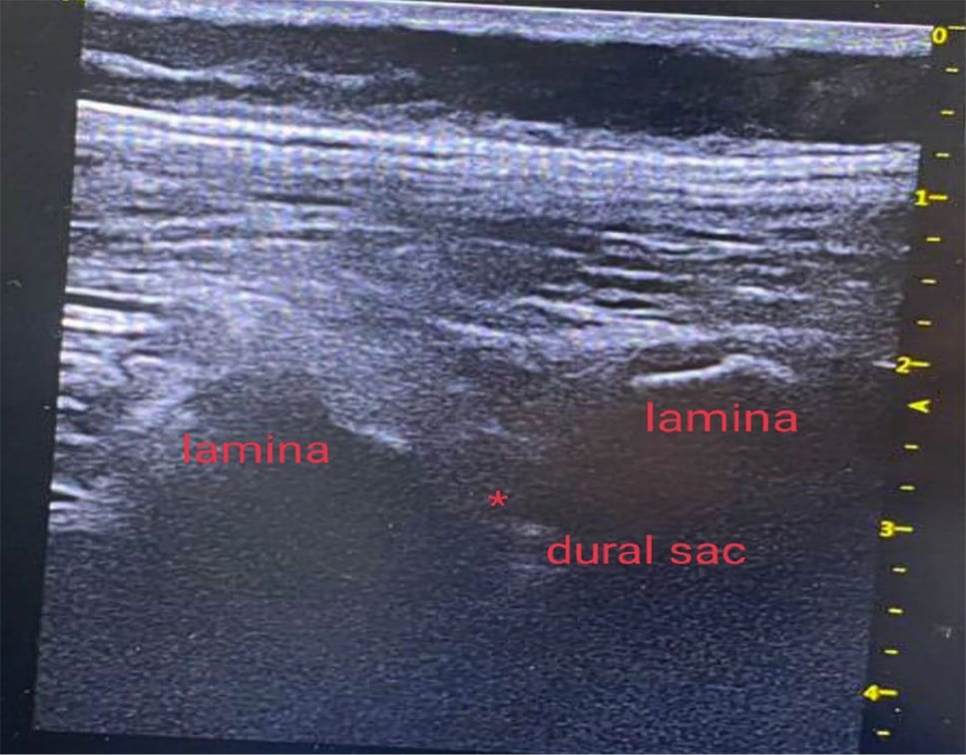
Ultrasound guided epidural block. _*_: site of injection
